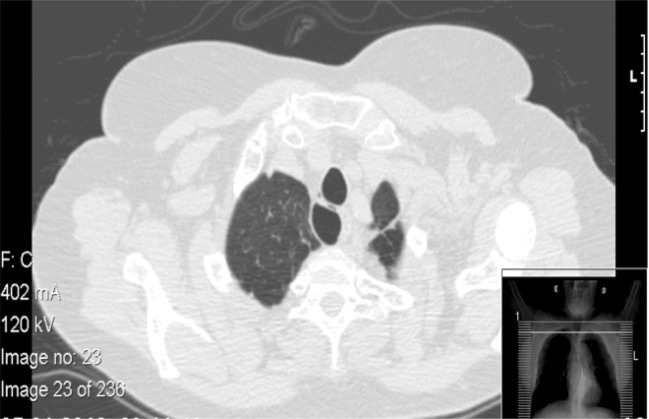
A CT scan of the chest at 10 days after admission, showing an air-filled multilocular mass (3.5 × 2.5 cm), with no evidence of infection, in the right wall of the trachea, near the apex of the lung.
